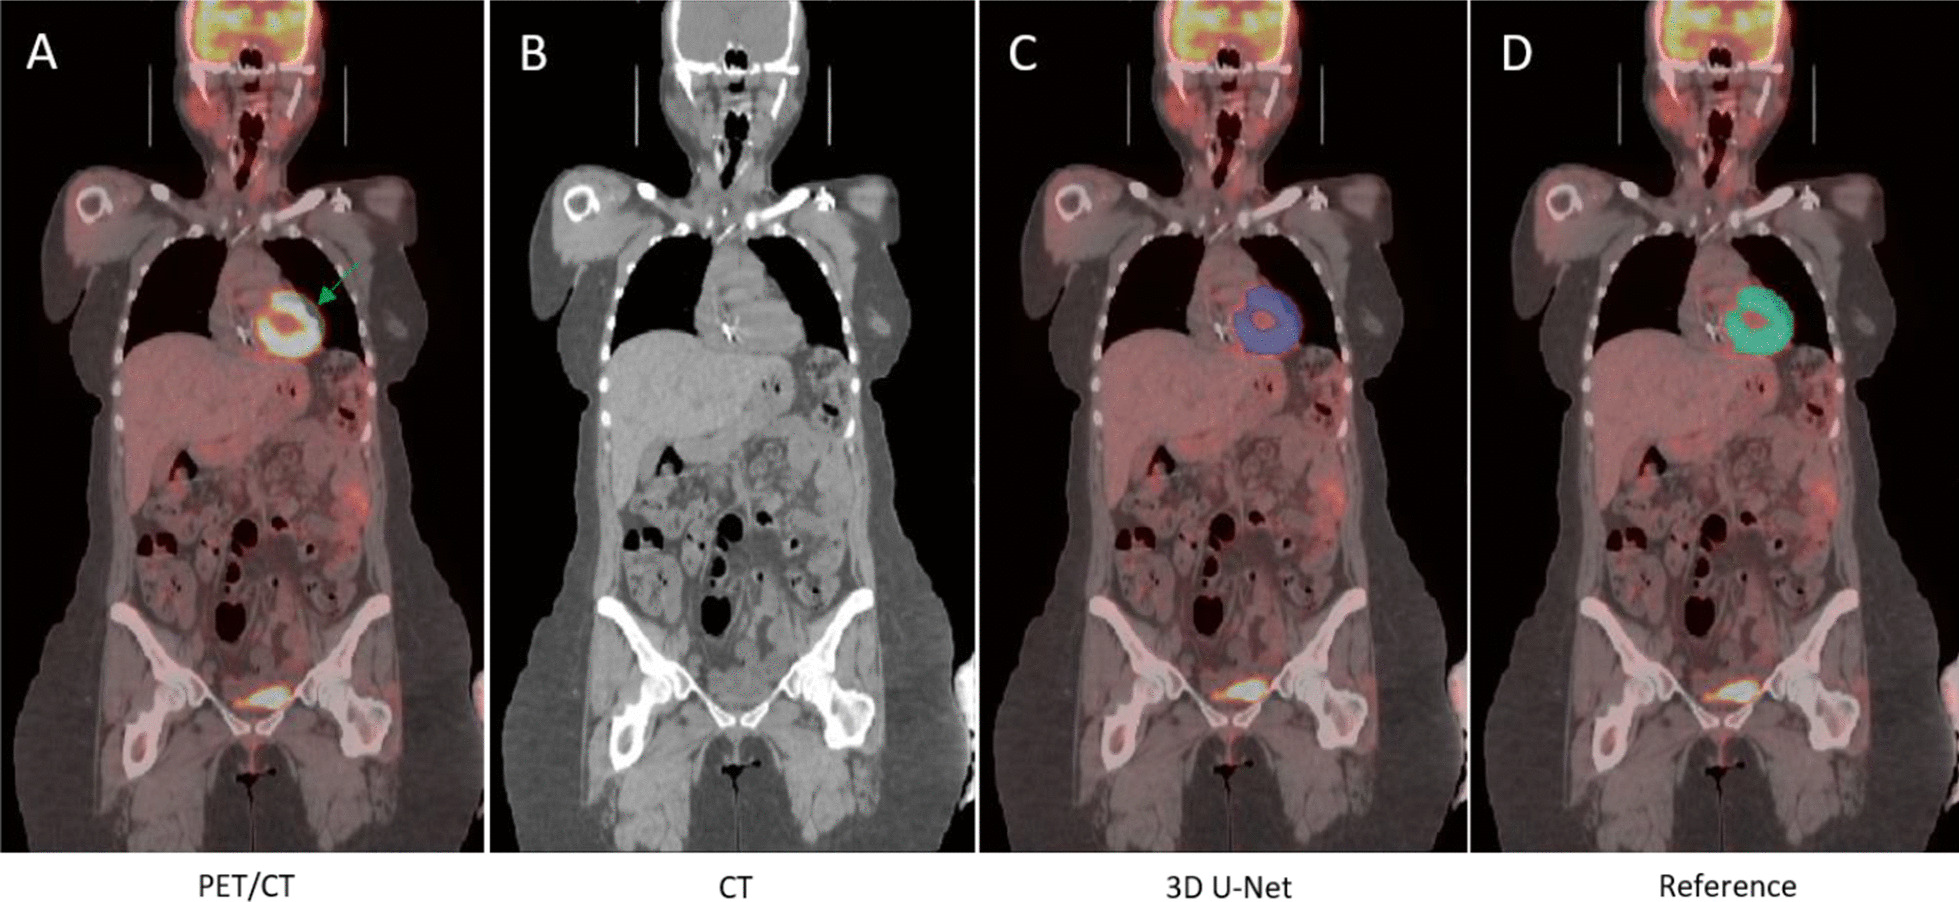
Example of heart segmentation on case where heart appears larger on PET than in the CT. a PET-CT, b CT, c heart segmentation by the model and d reference segmentation
